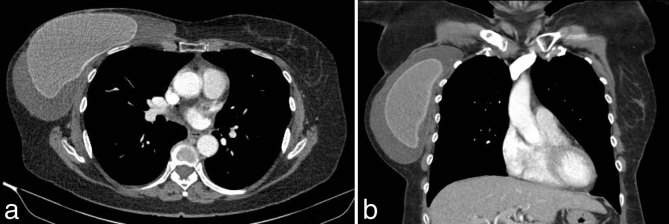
Pre-operative contrast-enhanced CT scan (a) axial and (b) coronal views demonstrating right mastectomy with implant and associated effusion in the implant cavity.
